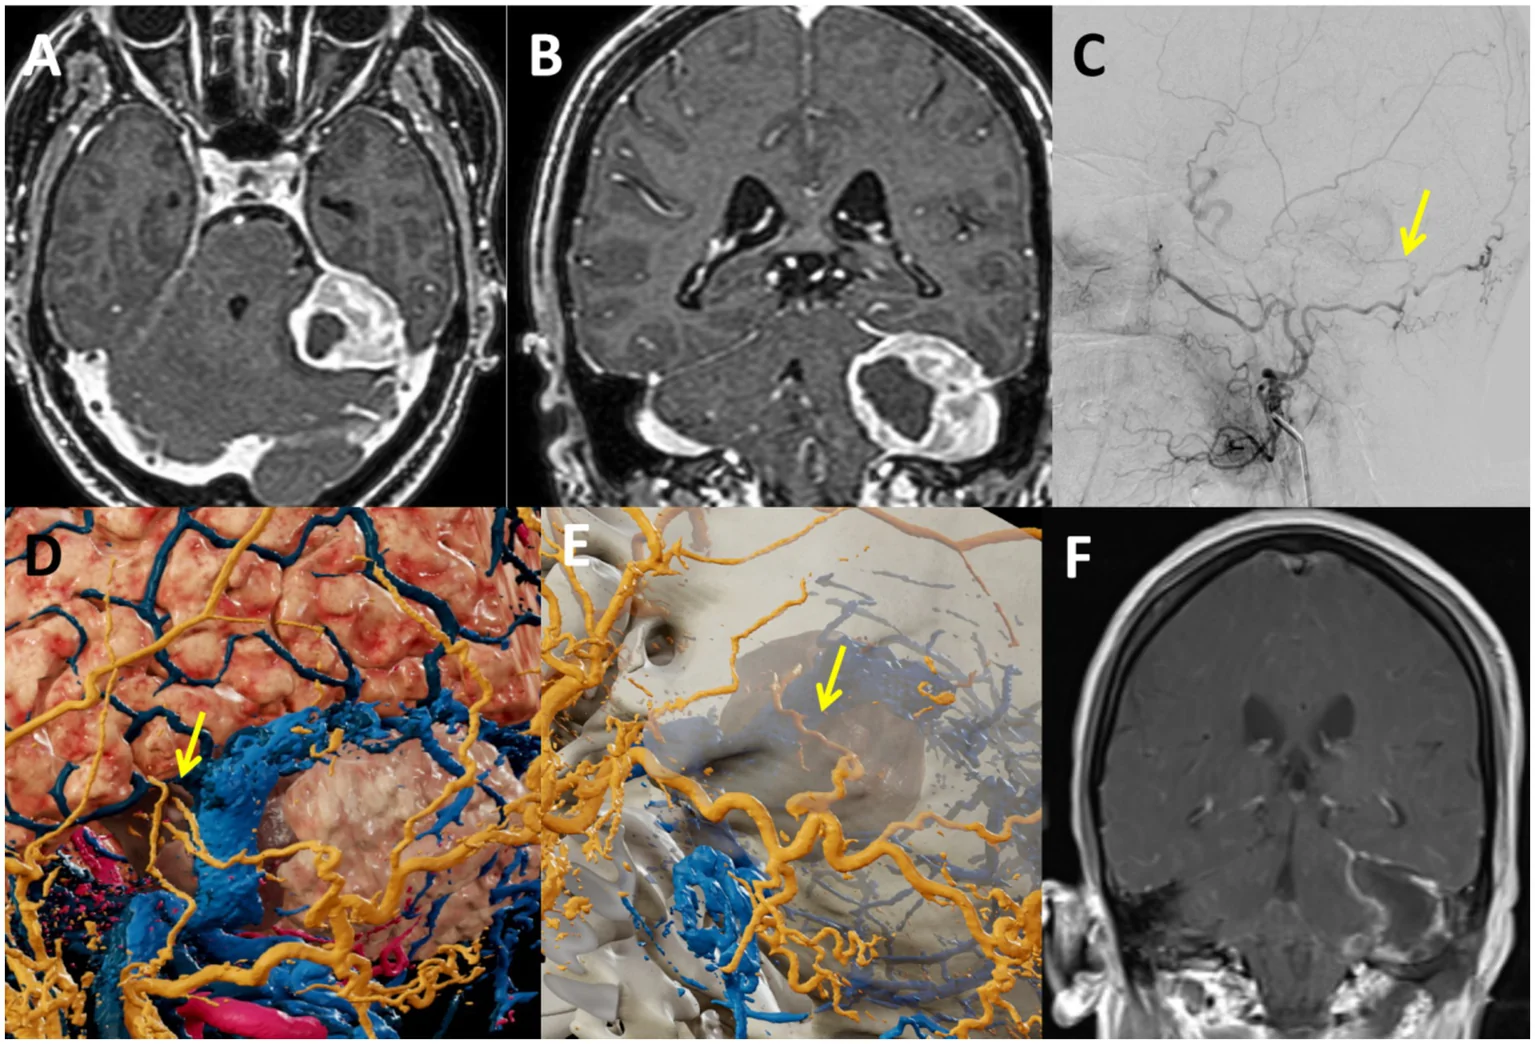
Tentorial meningioma supplied by a dural branch of the occipital artery. (A,B) Preoperative MRI showing a tentorial meningioma. (C) Preoperative DSA demonstrating tumor-feeding arteries originating from the dural branch of the occipital artery. The feeding arteries are indicated by yellow arrows. (D,E) MFI confirming that the feeding arteries traversed the petrous bone to reach the tumor base in the tentorial region, supporting selection of the presigmoid approach. (F) Postoperative MRI confirming Simpson grade I resection.
